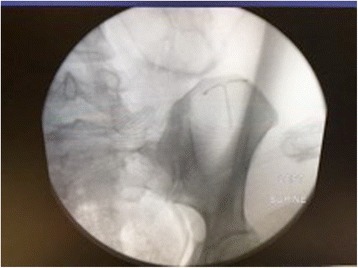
Intraoperative kidney-ureter-bladder X-ray with fluoroscopic guidance showing intrauterine device anterior to patient’s left acetabulum
